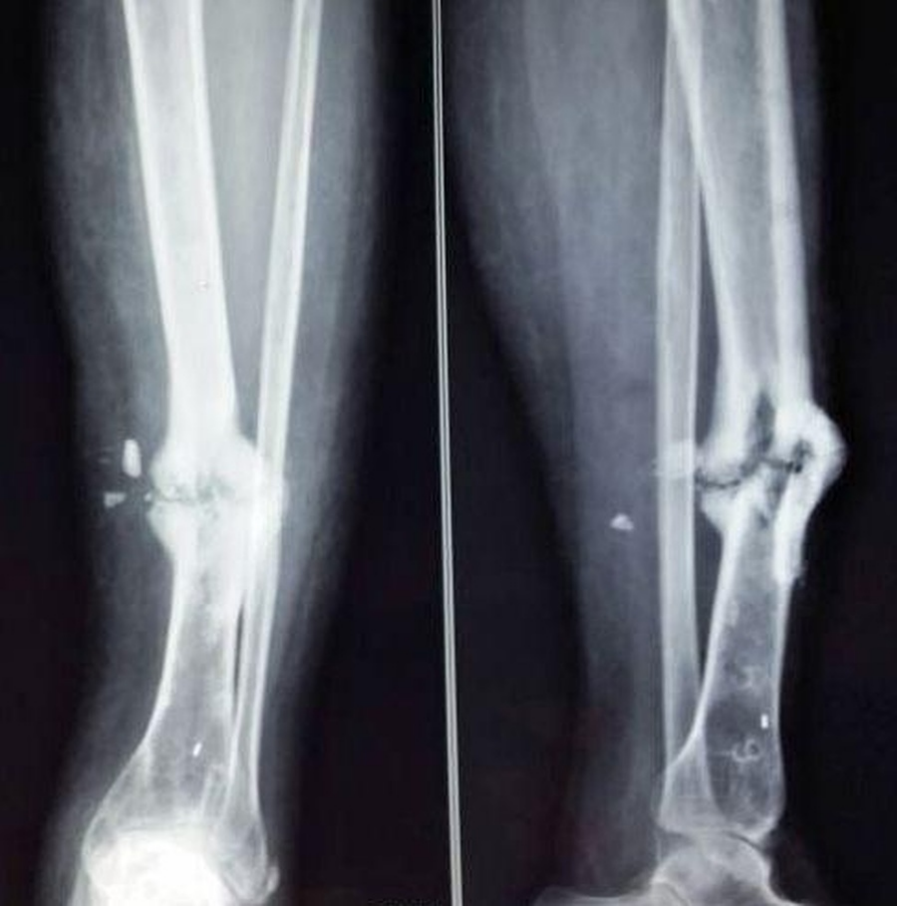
Preoperative radiographs showing nonunion of the tibia
